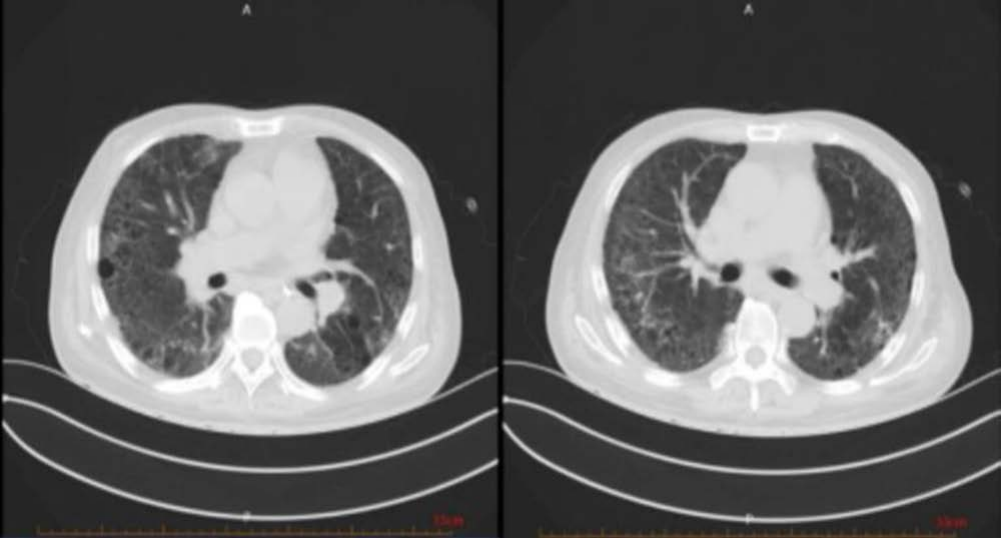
Lung CT on June 12th: compared with April 11th, multiple exudative changes in both lungs have decreased compared to before, and bilateral pleural effusion has basically disappeared compared to before.
